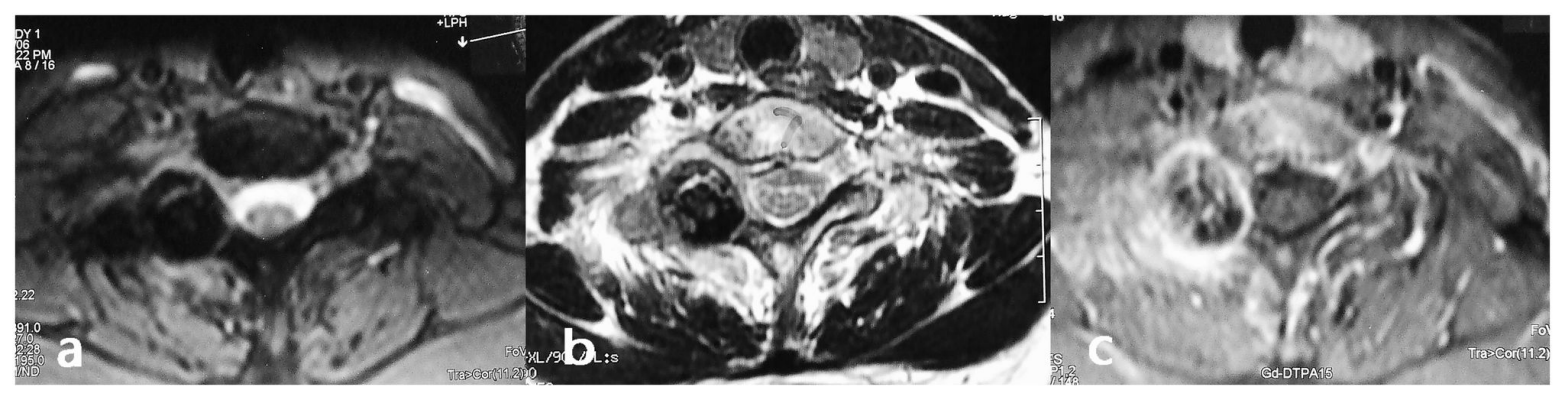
Preoperative MRI demonstrates single cystic lesion with cortical destruction at the posterior elements of C7 vertebra and slight edema of the surrounding soft tissues. Enhanced MRI shows a rich vascular supply to osteoid tissue, and the image has been enhanced significantly. a,b: T1 and T2 weighted image; c: enhanced MRI.
